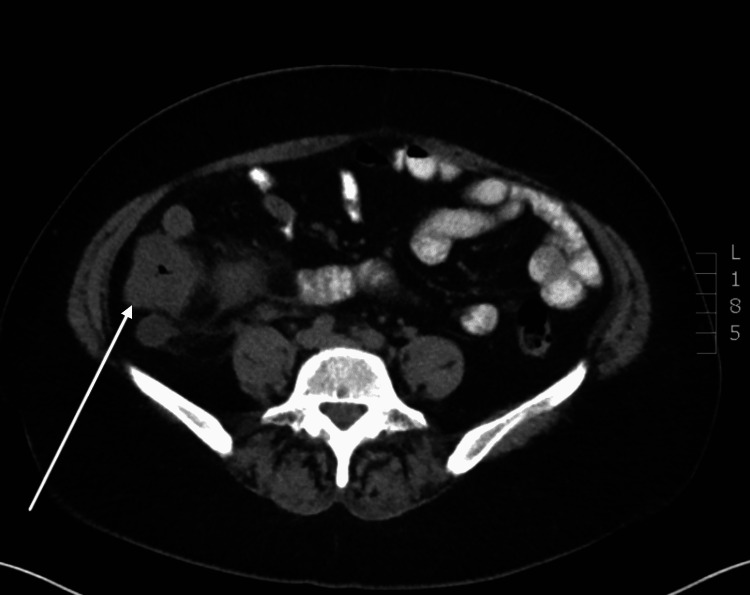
CT scan of the abdomen and pelvis with oral contrast (axial slice). Arrow indicating cecal thickening just posterior the engorged appendix. CT: computed tomography
